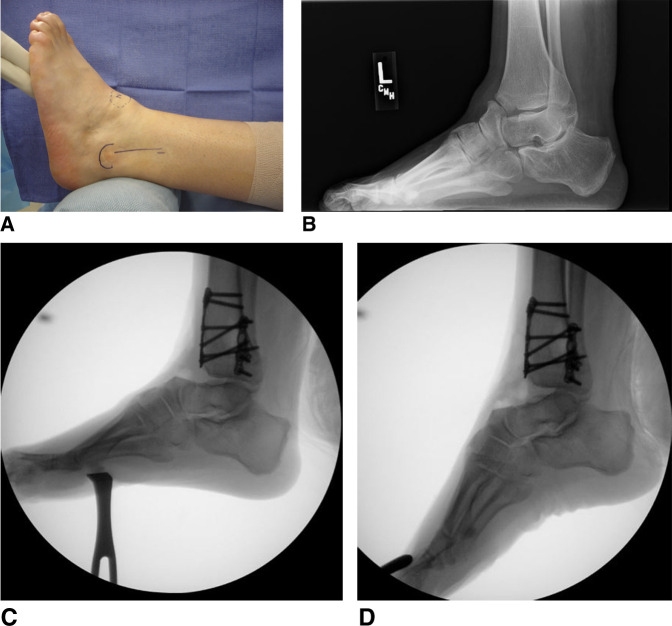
Anterior Impingement. A–D, Preoperative, intraoperative, and postoperative radiographs and preoperative clinical photographs of an 11-year-old boy with a history of congenital clubfoot and multiple previous surgeries. His primary report was anterior ankle pain and inability to achieve neutral dorsiflexion. Residual cavovarus deformity was also present. He was treated with a dorsiflexion-producing anterior closing-wedge tibial osteotomy with fibular osteotomy, midfoot osteotomy, plantar fascia release, and anterior tibialis tendon transfer to second cuneiform.
